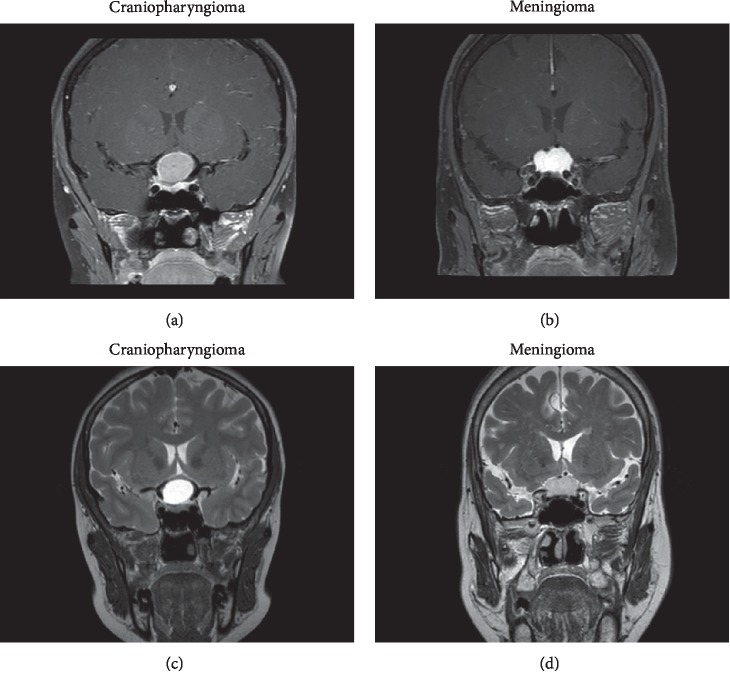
Examples of two cases from the MR images in patients with craniopharyngioma and meningioma. (a) Contrast-enhanced images with craniopharyngioma, (b) a contrast-enhanced image with meningioma, (c) images of T2WI with craniopharyngioma, and (d) an image of T2WI with meningioma.
